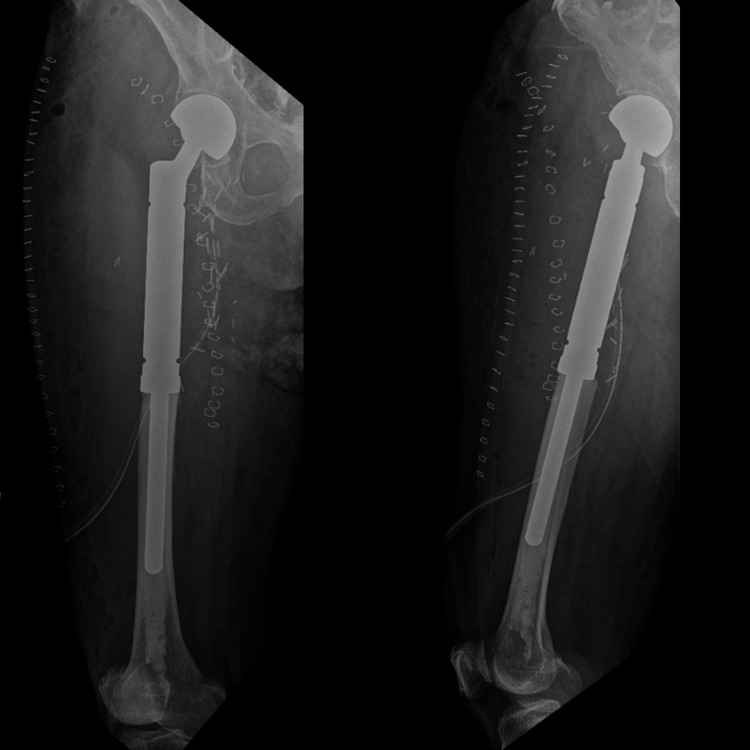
Post-operative plain radiography showing right proximal femur mega prosthesis
